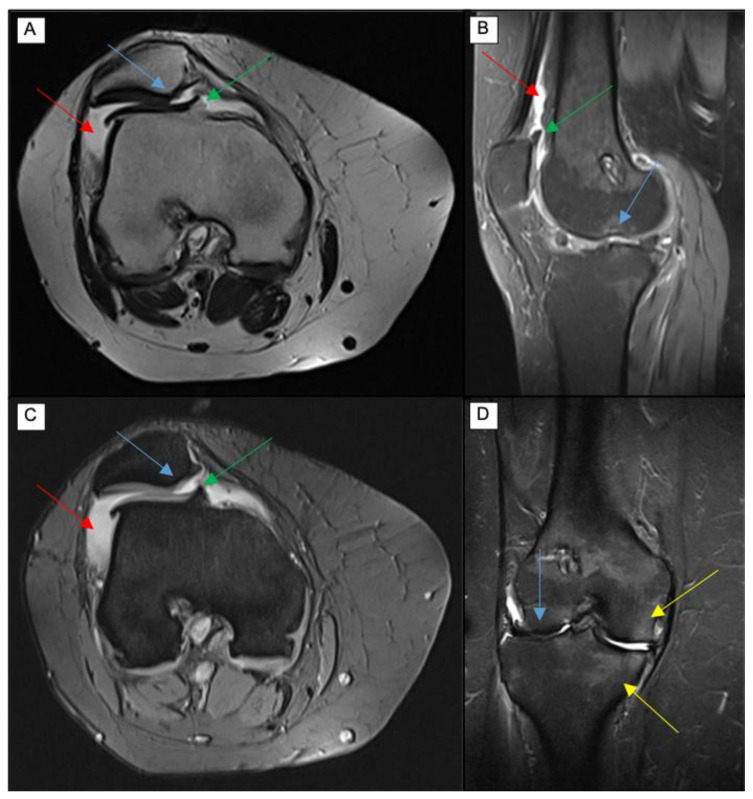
Axial T2 (A) and GRE (C), sagittal PD (B), and coronal STIR (D) MRI sequences showing features of severe knee osteoarthritis, including femoropatellar and femorotibial cartilage damage (blue arrows), effusion with synovitis (red arrows), marginal osteophytosis (green arrows), and subchondral bone marrow edema (yellow arrows).
